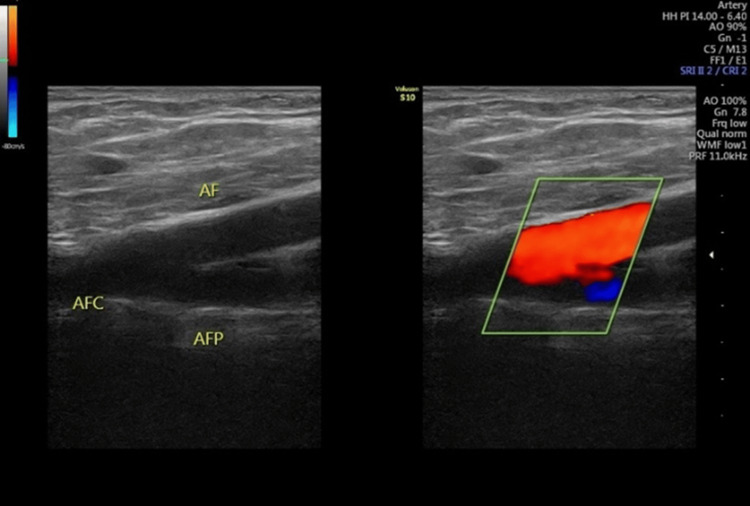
Doppler ultrasound of the common femoral artery, femoral artery, deep femoral artery, popliteal artery, anterior tibial artery, and posterior tibial artery with negative findings of arterial thrombosis. AFC: common femoral artery; AF: femoral artery; AFP: deep femoral artery.
